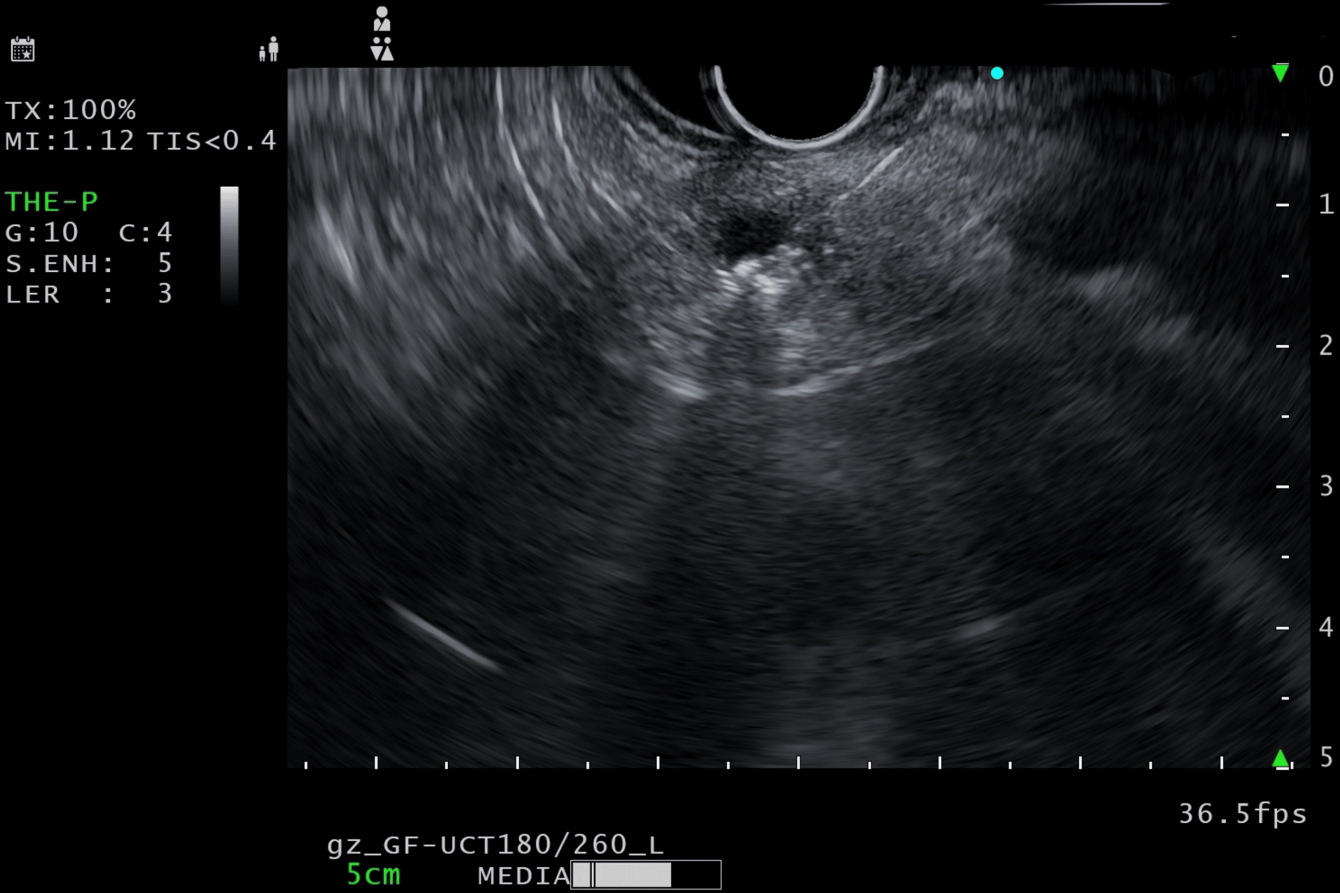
The EUSRA needle was introduced into the lesion EUSRA: Endoscopic ultrasound radiofrequency ablation
